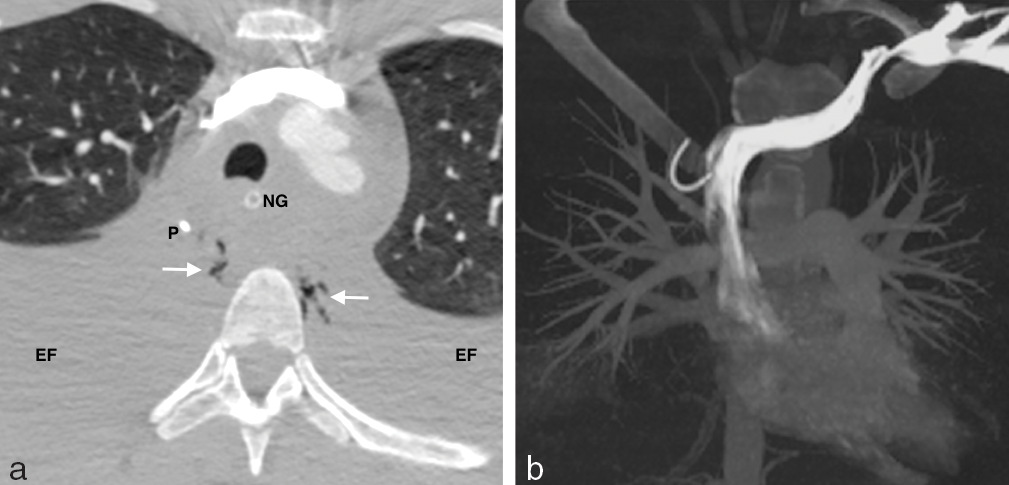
(a) Axial slice from the CTPA shows large bilateral pleural effusions (EF) and pneumomediastinum (white arrows). A nasogastric tube (NG) is sited within the oesophagus, which is displaced anteriorly together with the trachea by a large mediastinal effusion. The PICC tip (P) is adjacent to locules of mediastinal gas. The azygos is not clearly seen on this image, as it is isodense to the surrounding effusion. (b) Coronal three-dimensional maximum intensity projection reconstruction of the CTPA shows the PICC displaced outside the superior vena cava. CTPA, CT pulmonary angiogram; PICC, peripherally inserted central venous catheter.
